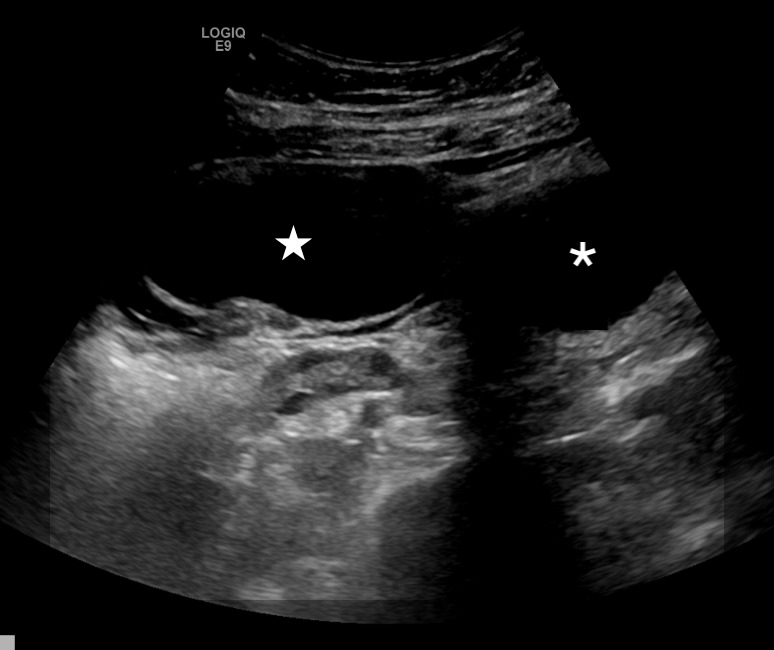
Transabdominal ultrasound image obtained with a curvilinear 5-megahertz transducer demonstrates a 7-centimeter cystic mass (star) in the right adnexa near midline, adjacent to the bladder (asterisk).
